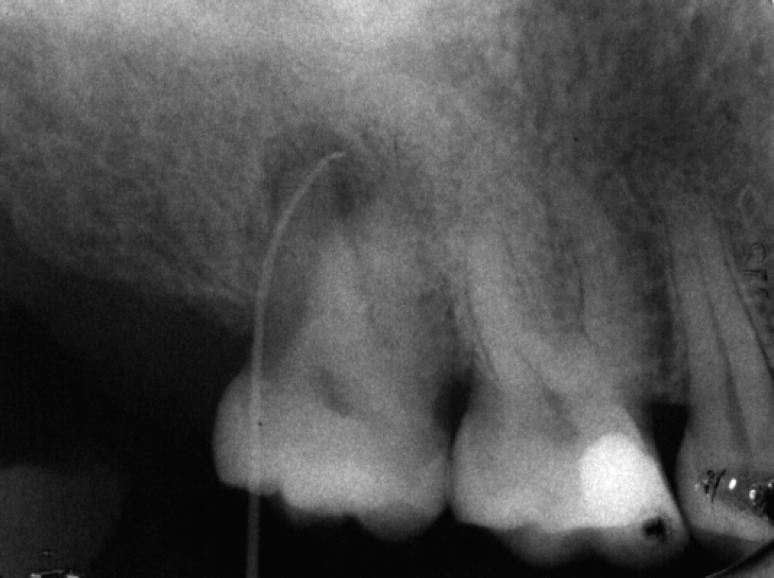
Tracing radiograph shows the origin of lesion is endodontic
